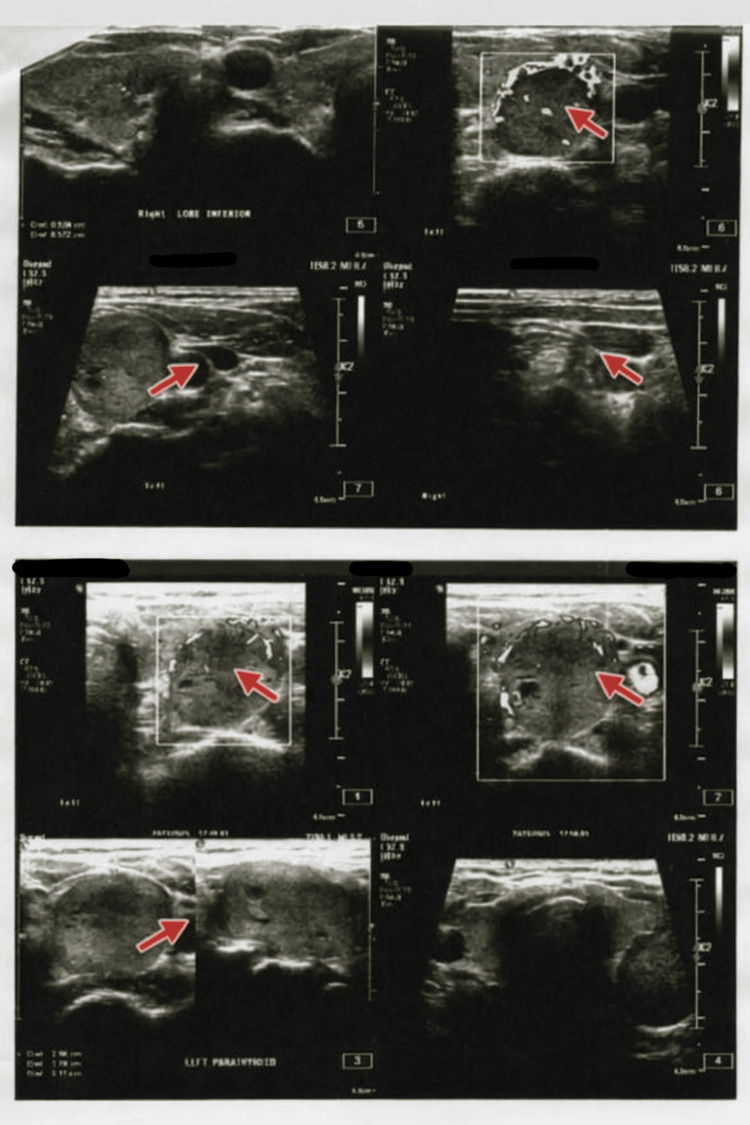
Ultrasound images of the left thyroid nodule.
